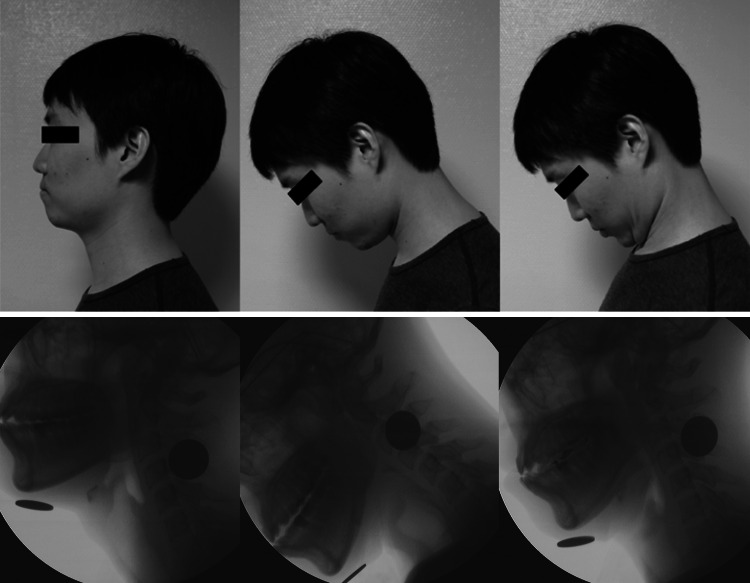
The instructions for the study postures (*upper pane*). The radiographic difference with each posture (*lower pane*)
